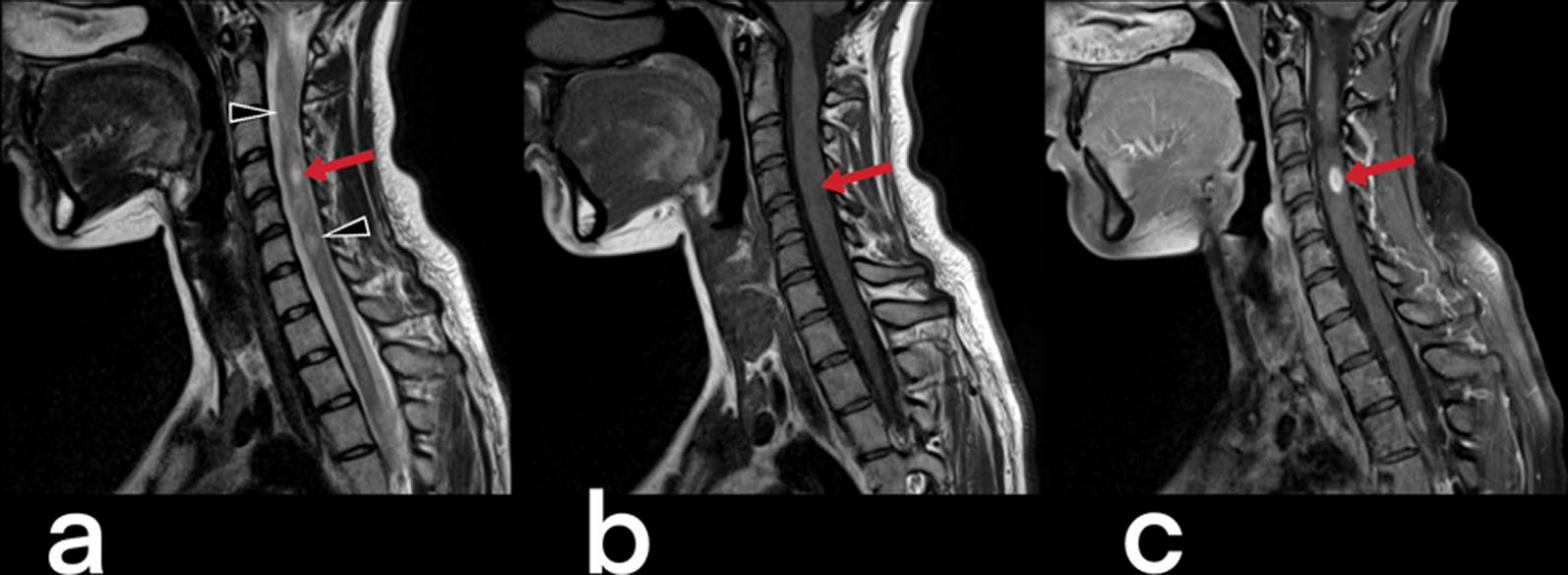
MR images of a 17-year-old female with the diagnosis of cervical tuberculous myelitis. Sagittal T2 weighted image (a) shows a heterogeneous increased signal (edema, inflammation) on the spinal cord (arrowheads) and focus (arrow). The cervical spinal cord is observed as an enlarged diffuse hypointense area on pre-contrast T1-weighted image (b). Post-contrast (c) T1 weighted image reveals diffuse heterogeneous and focal (arrow) contrast enhancement of the same region. The focus is a tuberculoma (arrows)
